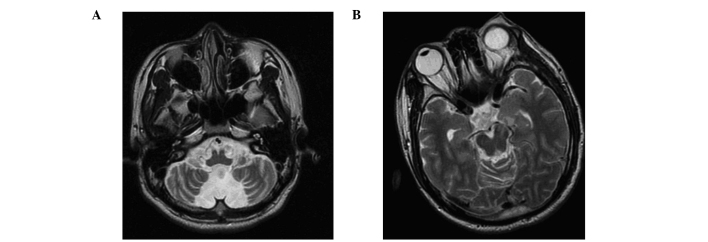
Comparison of MRI scans prior to and following robot assisted RFTC treatment. (A) Cerebellar hypoplasia was confirmed preoperatively. (B) Following robot assisted RFTC treatment, the volume of the right hippocampus still less than the left, the right temporal horn still larger than left and the target of RFTC treatment was the head of the left hippocampus. RFTC, radiofrequency thermocoagulation; MRI, magnetic resonance imaging.
